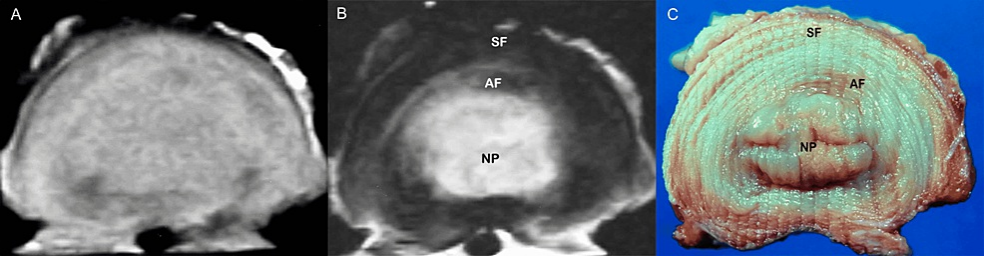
Normal disc, MR images and gross inspection. Axial T1-weighted (A) and T2-weighted (B) MR images show homogenous, intermediate signal intensity in the disc (A) and a central area of high signal intensity, the nucleus pulposus, surrounded by a peripheral portion of low signal intensity, the annulus fibrosus (B). (C) Axial anatomic section demonstrates the internal structure of the normal disc. Centrally located area of high signal intensity corresponds to the nucleus pulposus and the inner portion of the annulus fibrosus. NP: nucleus pulposus, AF: annulus fibrosus, SF: Sharpey fibers.
